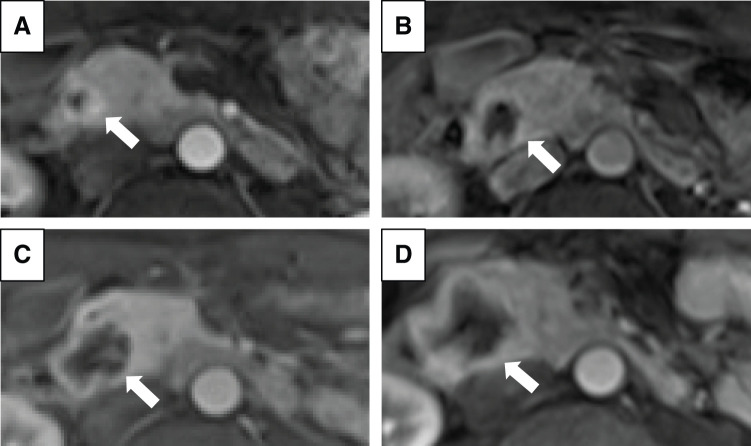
Time-dependent increase in the pancreatic tumor size on MRI. (A) 18 months before surgery (12 mm); (B) 12 months before surgery (22 mm); (C) 6 months before surgery (28 mm); and (D) immediately before surgery (33 mm). White arrows indicate the tumor.
